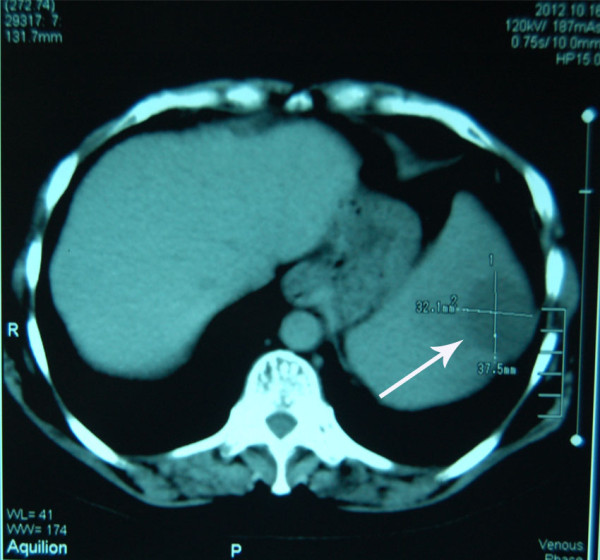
Abdominal computed tomography showing the space occupying lesion inside the spleen (arrow).
